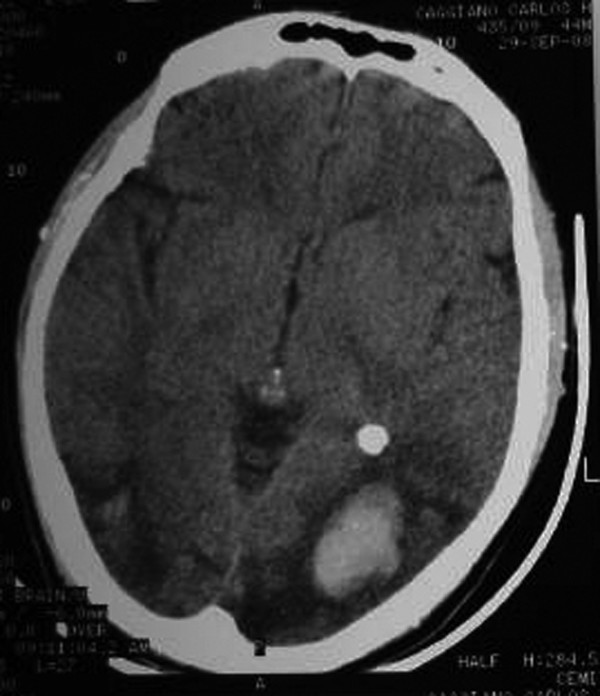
Brain CT without contrast bilateral occipital cortico-subcortical hypodensities and hemorrhagic foci.
